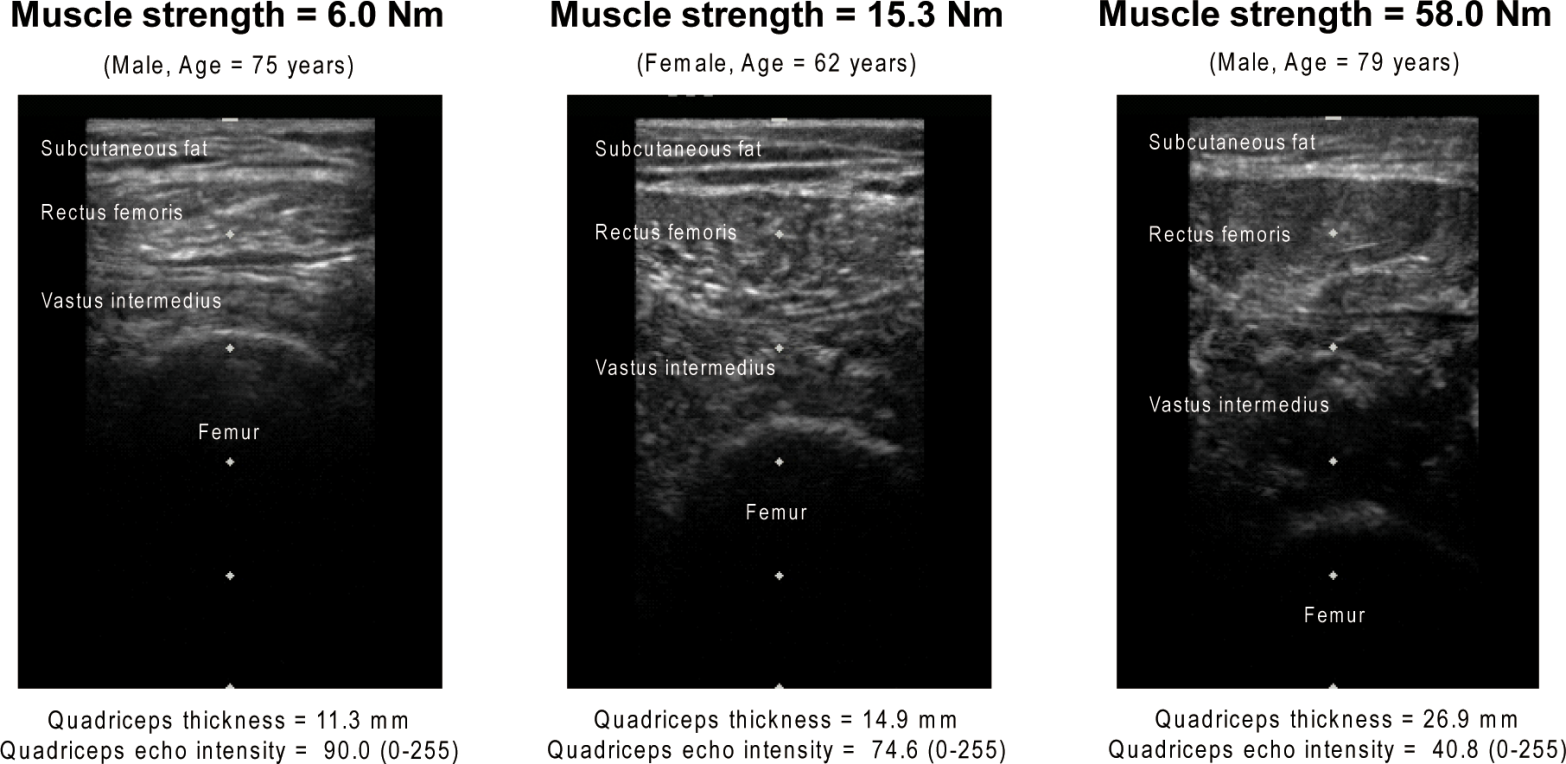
Typical ultrasound images of the paretic lower extremity.
